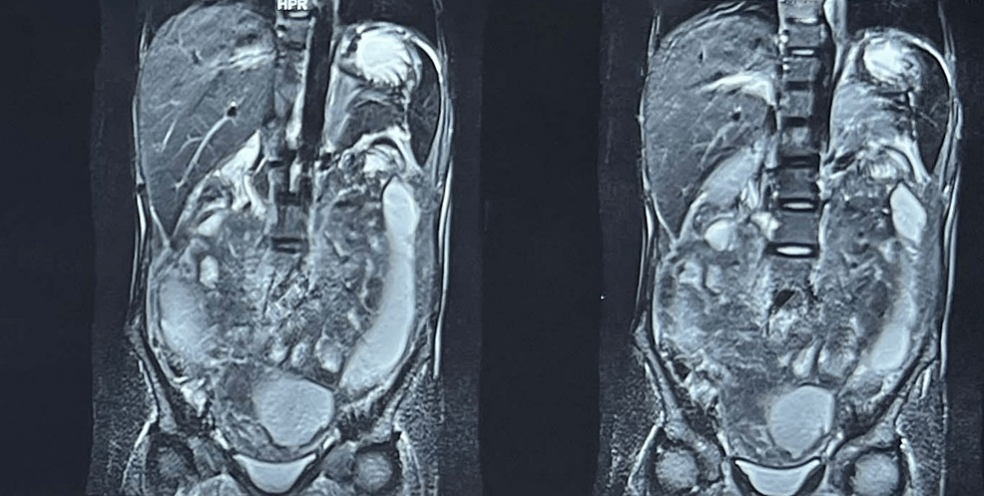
MRI of the abdomen showing signs of hollow viscus perforation
